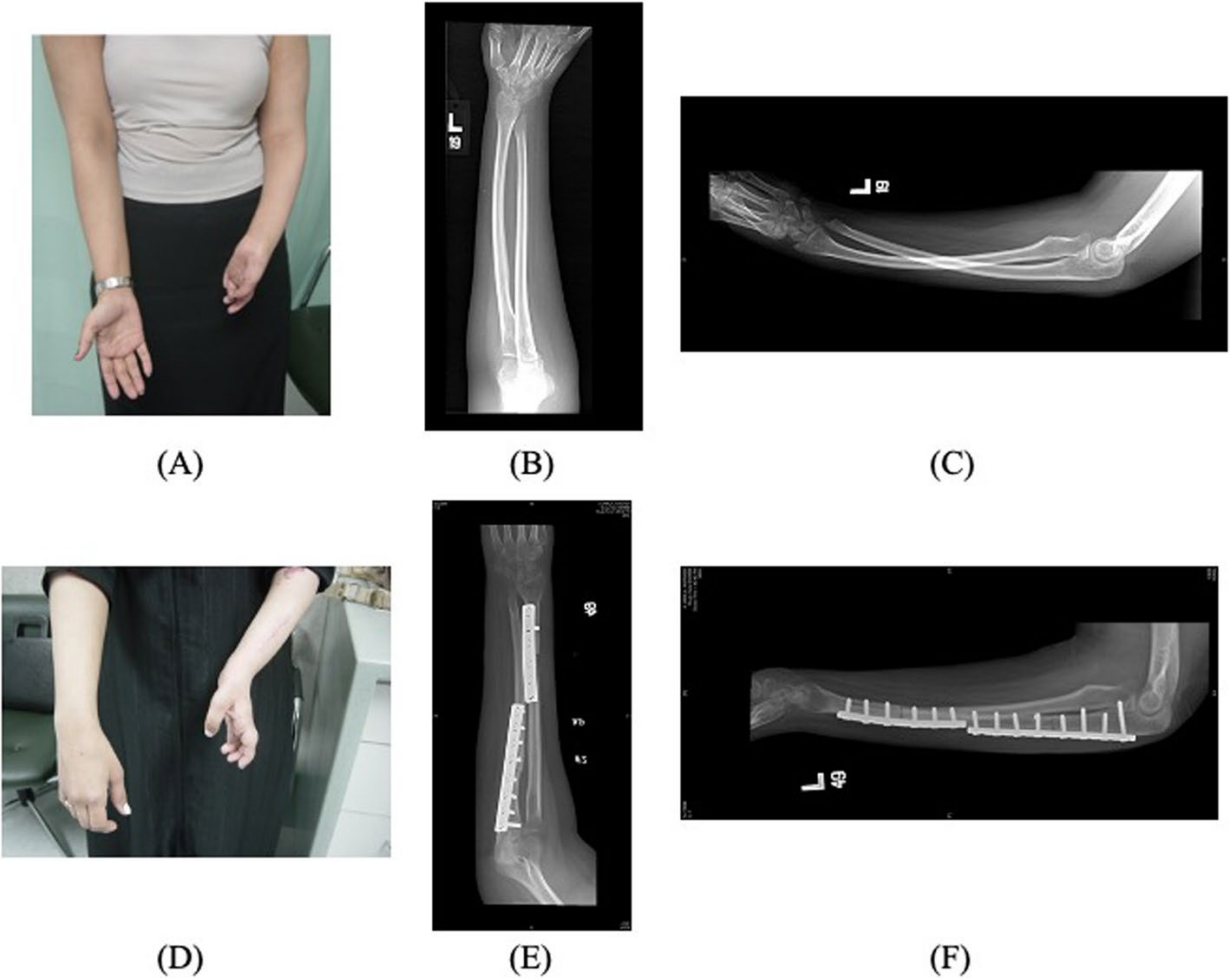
A 25-year-old female with OBPP sequelae with supination deformity of the forearm underwent forearm pronating osteotomy. (a) Left forearm resting position preoperatively, 90° (b) AP radiograph showing incongruent distal radial ulnar joint (DRUJ). (c) Lateral radiograph showing incongruence of DRUJ and bowing of the ulna (d) Three months postoperatively, the resting position changed from 90 supinations to 20° of pronation postoperatively (e) AP radiograph showing the osteotomy is satisfactorily closed, and the proper position length of the screw (f) Lateral view, the length of the screw is adequate and in the proper position
